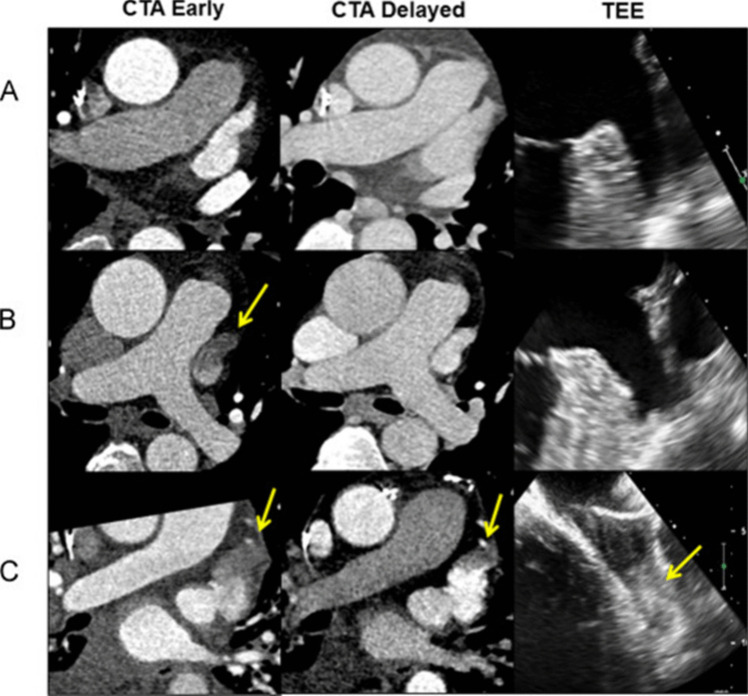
Cardiac Computed Tomography and Transesophageal Echocardiogram for Evaluation of Left Atrial Appendage Thrombus.Three examples of left atrial appendage thrombus evaluations by early and delayed computed tomography angiography (CTA) and transesophageal echocardiogram (TEE). Normal early and delayed filling of left atrial appendage (A) is a negative finding for thrombus. A left atrial appendage filling defect (*yellow arrows*) is also negative if it resolves on delayed imaging (B), otherwise it is positive if the defect has a typical appearance of a thrombus (C). Adapted from Bilchick et al. Heart Rhythm, 2016. 13(1):12–9, with permission from Elsevier [100]
